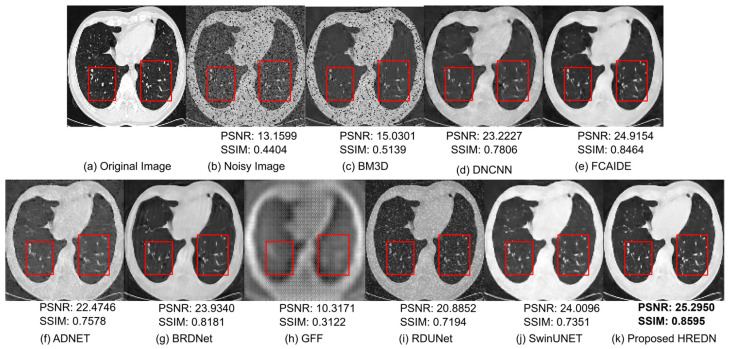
Visual comparison results for eight CT scan image denoising methods on single testing image from Curated COVID CT dataset with noise level (G:30 + RSP).
